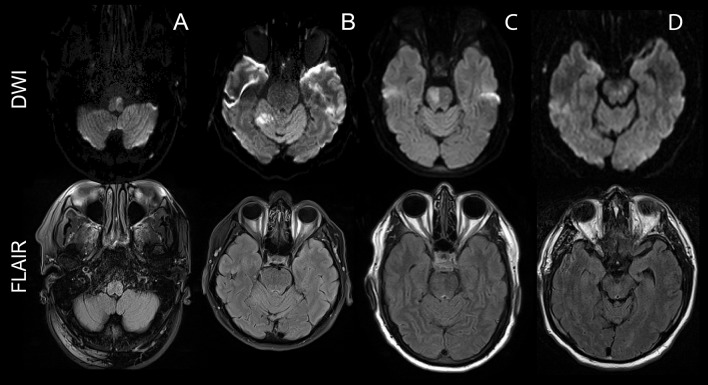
Examples of infratentorial strokes in the intention-to-treat WAKE-UP cohort. The upper row shows diffusion-weighted images depicting the acute ischemic stroke. The bottom row shows a FLAIR image of the corresponding slice, depicting a lack of signal hyperintensity in the area of the acute stroke. The different columns offer examples for the different stroke locations included into this substudy: (A) depicts a stroke of the ventral left portion of the medulla oblongata, (B) a right-sided cerebellar stroke in the feeding territory of the superior cerebellar artery, (C) a right-sided stroke in the pons, and (D) a focal mesencephalic stroke.
